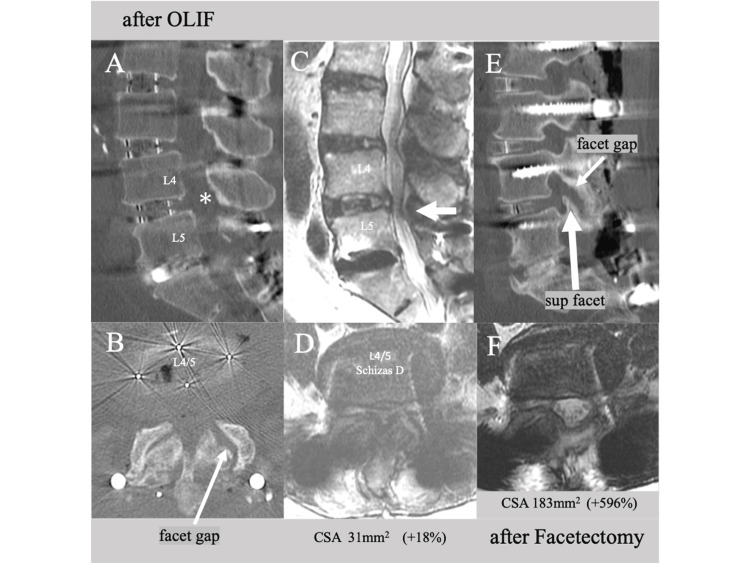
Postoperative CT and MRI images of Case 2 CT images taken immediately after OLIF showing (A) enlarged posterior disc height (asterisk) and (B) increased facet gap (arrow) at L4/5. Postoperative MRI images showing a slight increase in (C) the anteroposterior diameter of the dural sac (large arrow) and (D) CSA (an 18% increase). A parasagittal CT scan after the operation showing (E) residual lateral recess and foraminal stenosis due to a deformed facet at L4/5 (large arrow), despite an increased facet gap (arrow). An axial MRI image taken after a left L4/5 facetectomy (F) showing complete decompression of the L4 and L5 nerve roots and the dural sac. OLIF: oblique lumbar interbody fusion, CSA: cross-sectional spinal canal area
